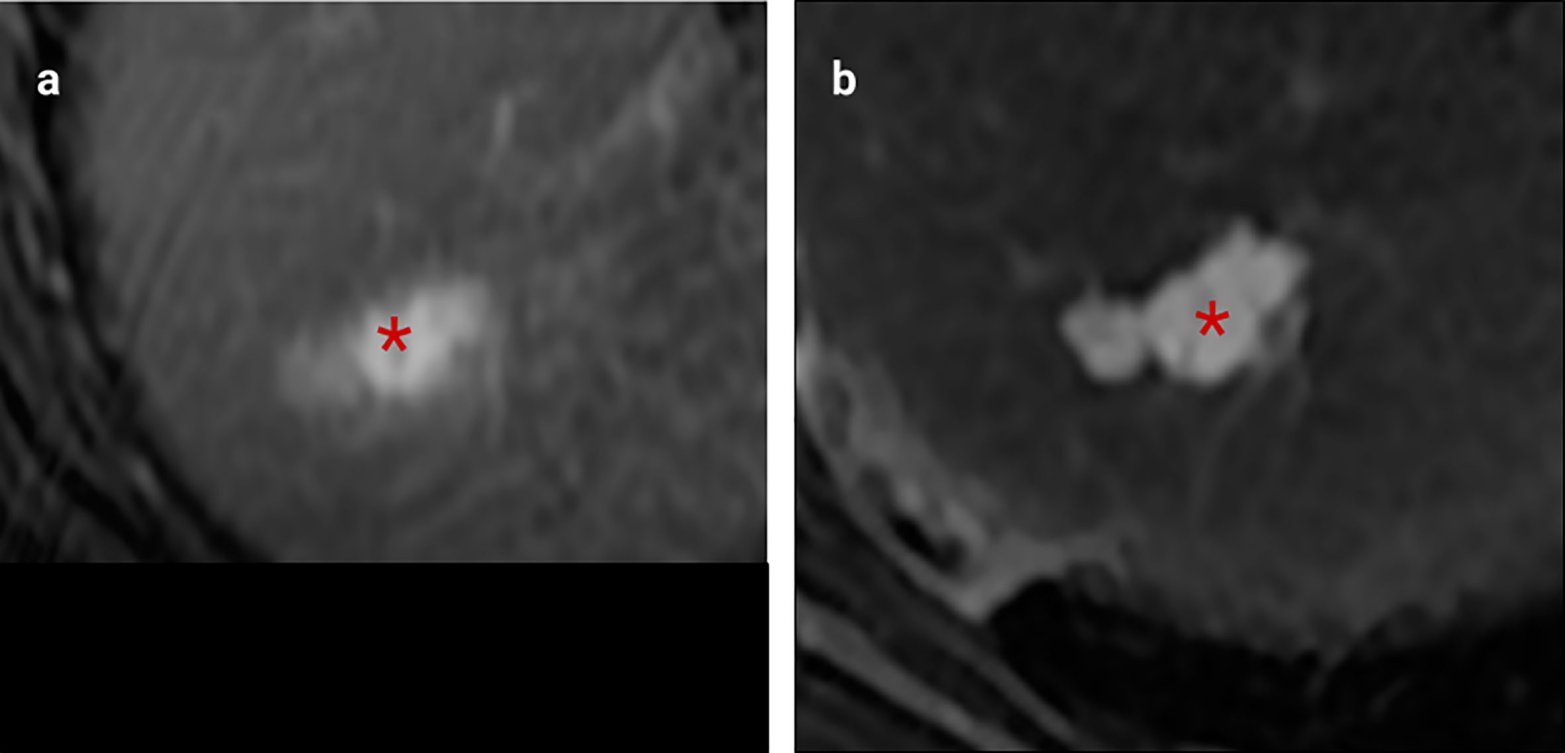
Example of excellent morphological response post-chemotherapy. Both images were obtained using the same MRI scanner performed at 1.5T (GE HDX Twinspeed MR scanner; GE, Milkwaukee, WI) with an 8-channel torso coil. (A) T2 weighted MRI showing a poorly defined CRLM with high T2 region of mucin (asterisk). (B). Following neoadjuvant treatment, this lesion demonstrates no change in size but now has a well-defined margin and replacement of intermediate signal cellular tumour with mucin, indicative of a good morphological response (asterisk). There was a complete pathological response at resection.
